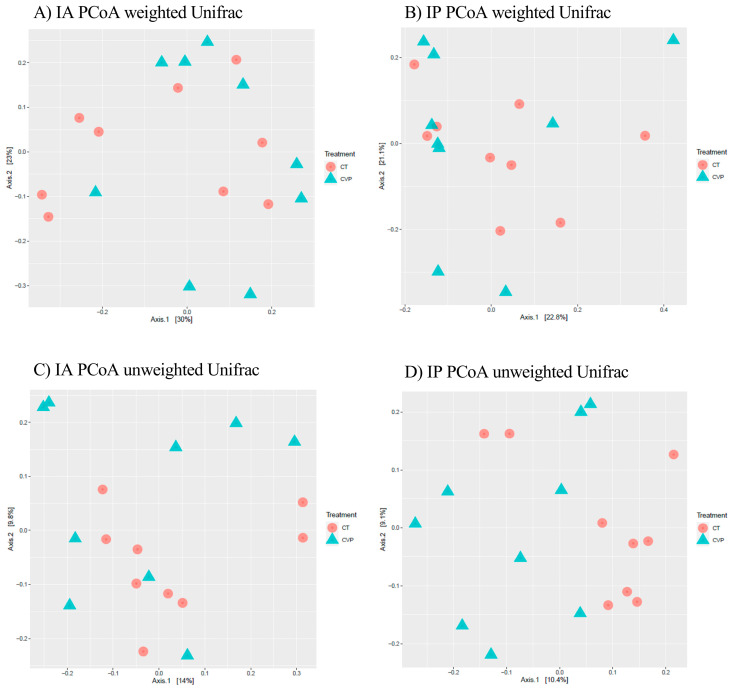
Principal coordinate analysis (PCoA) of bacterial community composition based on weighted and unweighted UniFrac distances. Circles and triangles represent samples from the intestinal anterior and posterior sections, respectively. In red and blue (circles and triangles) are shown samples from juvenile *C. labrosus* fed control (CT) and *C. fusca + V. proteolyticus* (CVP) diets for 90 days, respectively: (A) IA PCoA weighted Unifrac; (B) IP PCoA weighted Unifrac; (C) IA PCoA unweighted Unifrac; (D) IP PCoA unweighted Unifrac.
